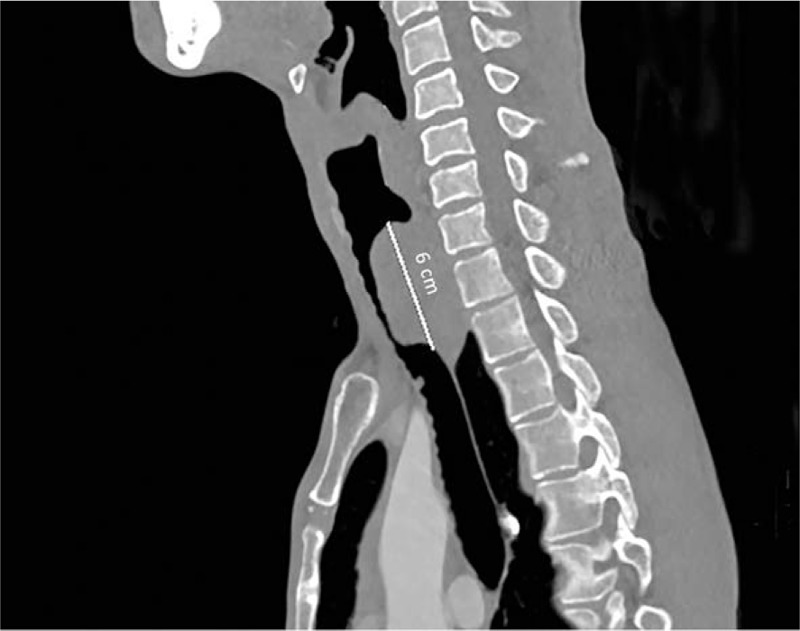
CT scan: occupying lesion in the cervical trachea, the pedicle was in the tracheal membrane, total length was about 6 cm.
